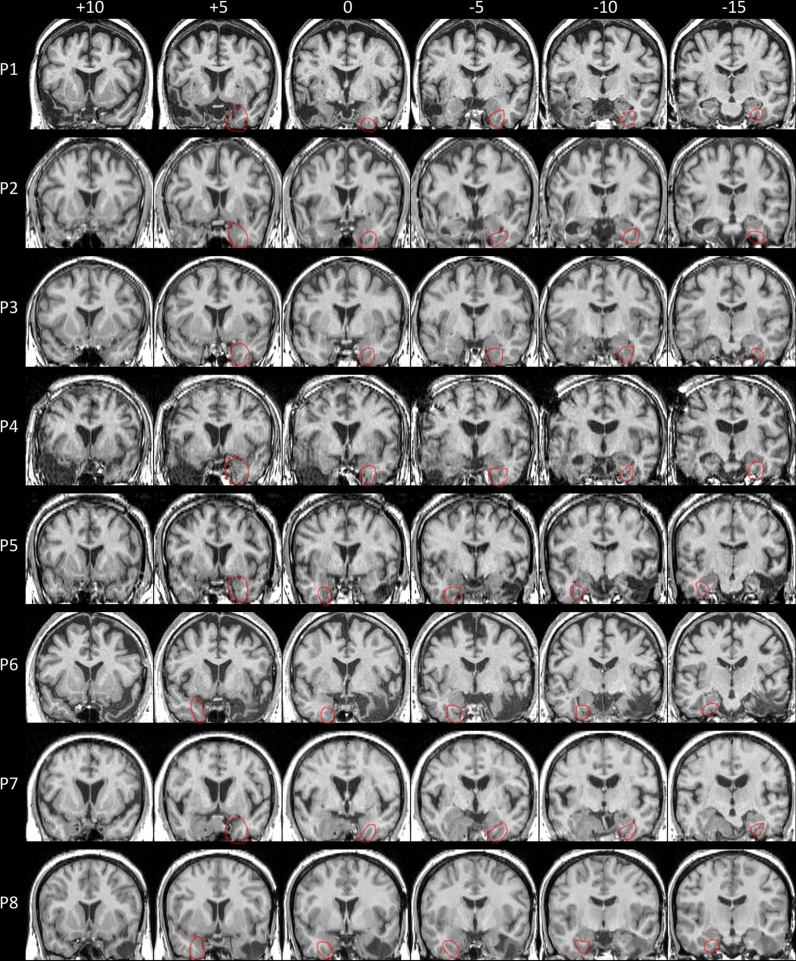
The vATL-damaged patients' lesions shown on T1-weighted structural scans normalised to MNI space. The perirhinal cortex in the intact, contralesional hemisphere is indicated in red. See Table 1 for lesion descriptions. MNI *y* and *z* coordinates are reported above each section. Images are shown in neurological convention with patient's left on image left.
